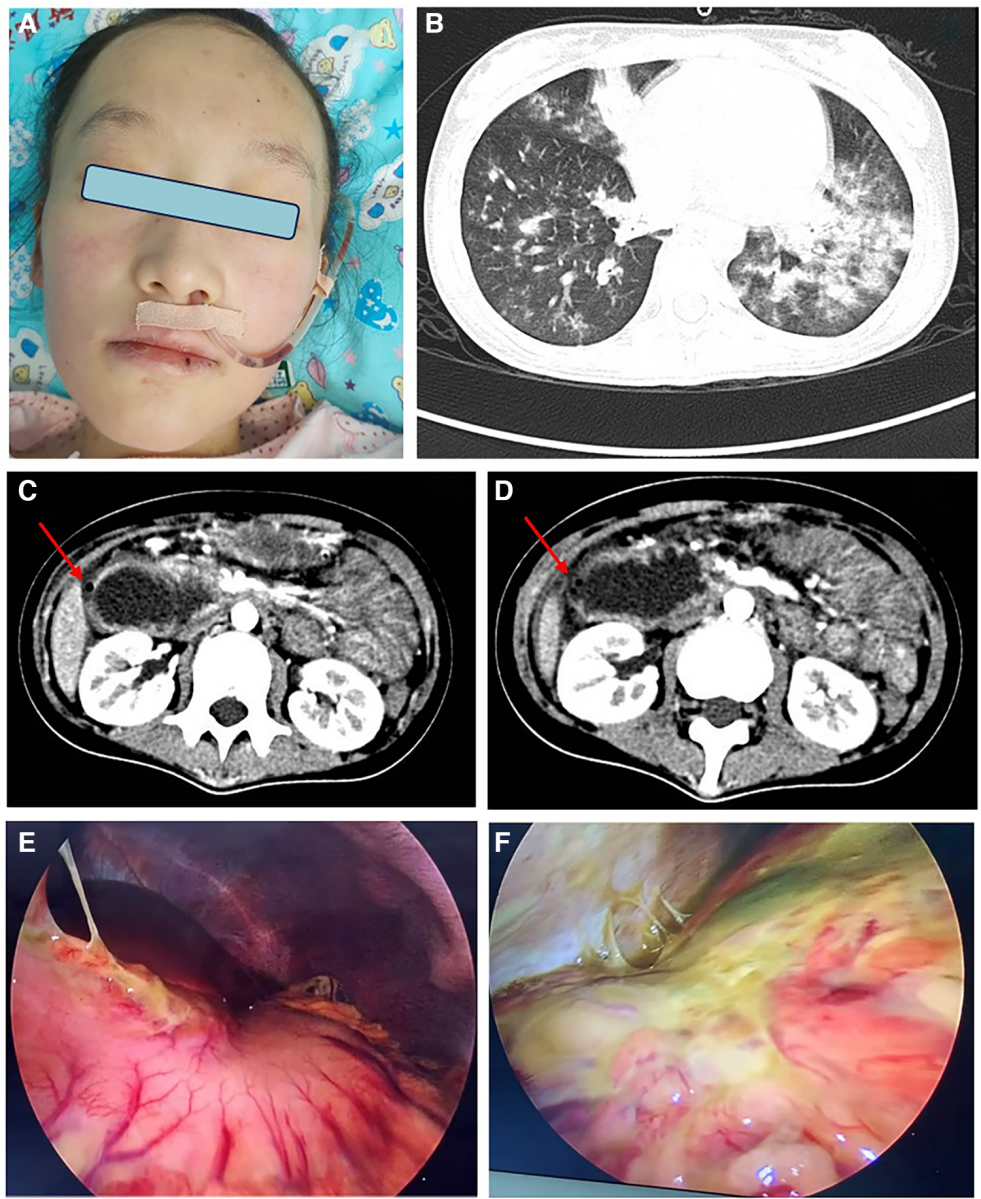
(A) Red patches and papules on the cheeks of this patient, via nasogastric decompression tube; (B) CT scan showed multiple patchy and patchy high-density shadows in both lungs; (C, D) CT scan of duodenal obstruction with perforation, intestinal wall edema is the site of perforation; (E, F) laparoscopic duodenal perforation with abdominal fluid accumulation and surface purulent secretion coverage.
